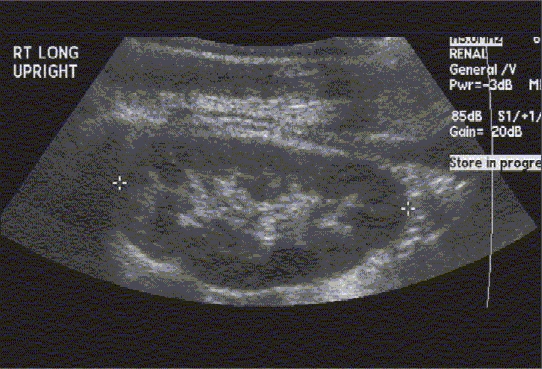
Renal Ultrasound, Lasix Renogram and Retrograde Pyelogram Patient is a 14-month-old infant born with prenatally detected hydronephrosis. Postnatal evaluation demonstrated mild left hydronephrosis. Serial US demonstrated progressive left hydronephrosis (b). Lasix Renogram demonstrated a significant increase in the T1/2 drainage of the left renal pelvis (102 min) (c). A left retrograde pyelogram demonstrates obstruction of the left UPJ (d). (a) US demonstrating normal right kidney
